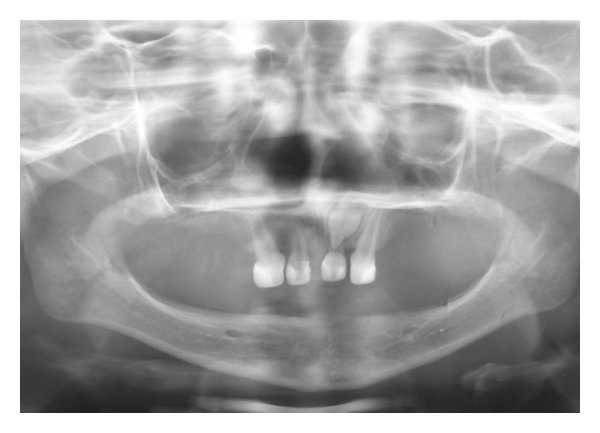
Panoramic radiograph showing oligodontia and lack of alveolar bone development.
